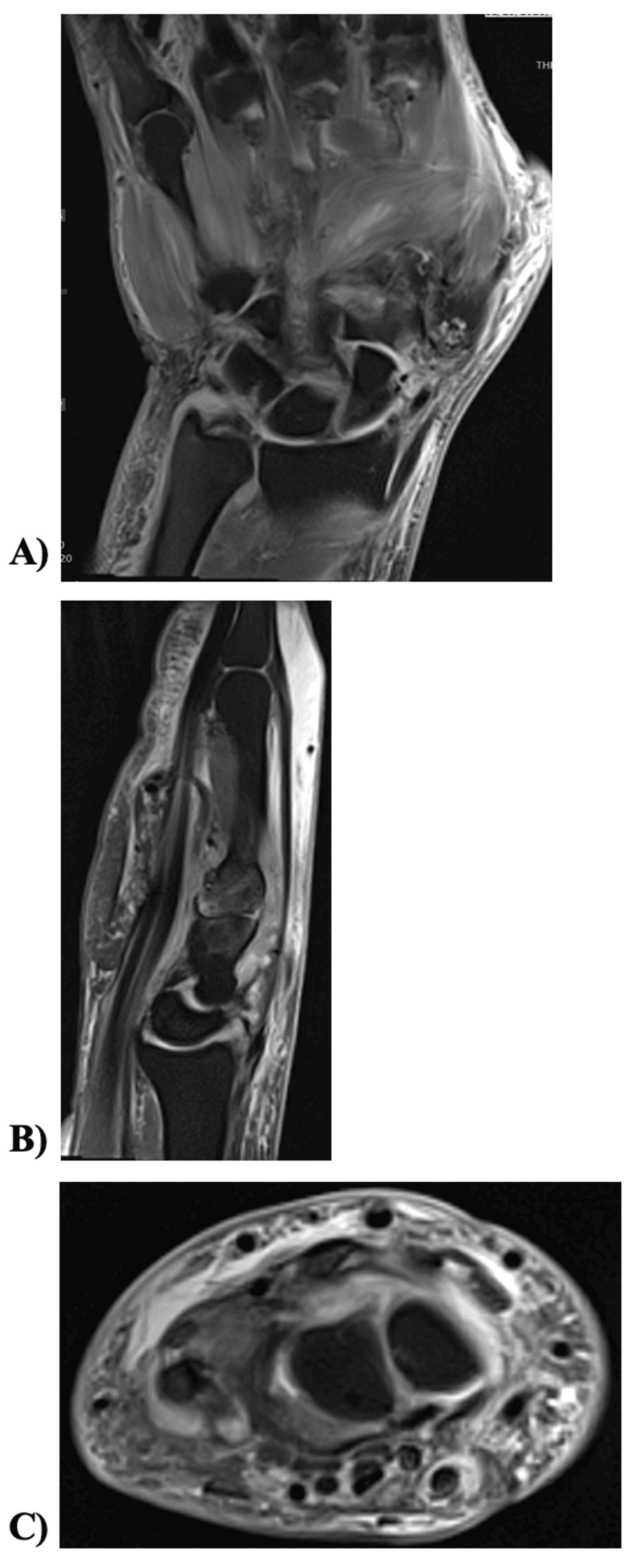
Initial left wrist MRI. (A) Coronal view showing diffuse muscle edema but no abnormal widening of the scapholunate interval or osteomyelitis. (B) Sagittal view view of the left wrist showing subcutaneous soft tissue swelling and edema throughout the hand and wrist with diffuse muscle edema. (C) Axial view showing small 14x7 mm and 7x5 mm intramuscular fluid collections in the opponens digiti minimi.
